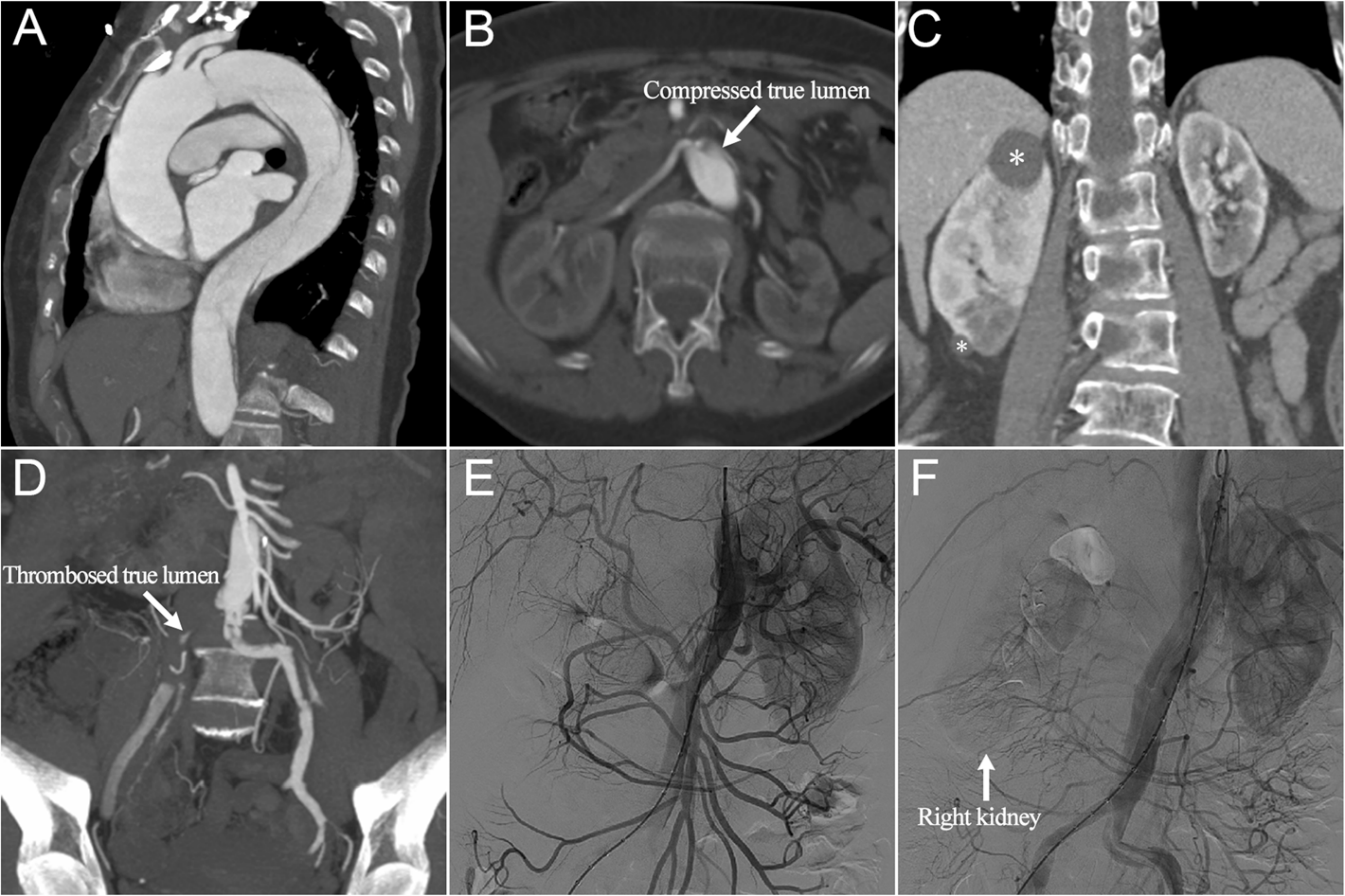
(a) Preoperative sagittal computed tomography angiography showed type B aortic dissection. (b) Left renal artery originated from a severely stenotic true lumen, right renal artery with dynamic occlusion was supplied via a false lumen. (c) Two cysts (asterisks) were separately located at the upper and inferior poles of right kidney. (d) Incomplete thrombosis was detected in the bilateral common iliac arteries. (e) Abdominal angiography showed poor perfusion of the right kidney and bilateral iliac arteries, and (f) completion angiography demonstrated the reopening of the distal true lumen and an improved flow in right renal artery and bilateral iliac arteries
